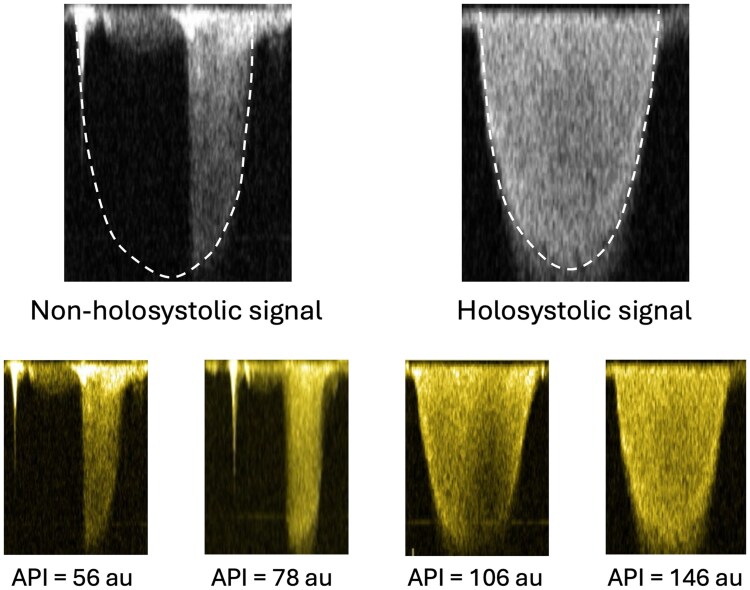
The average pixel intensity of the continuous-wave Doppler signal in mitral regurgitation. In the left upper panel, a parabolic tracing is traced, guided by the onset at the mitral closure click and extrapolated to the expected/estimated parabolic shape that includes the CWD signal to capture the entire systolic duration. Hence, the tracing includes the complete regurgitant flow variation in time; this tracing is more obvious for the holosystolic MR jet in the upper right panel. In the left lower panels, two telesystolic CWD signals typical for mitral valve prolapse are shown with relative low API values (56 and 78 au), whereas the lower right panels show two holosystolic MR jets with an API value of 106 au (moderate MR) and 146 au (severe MR), respectively (see also text for explanation).
